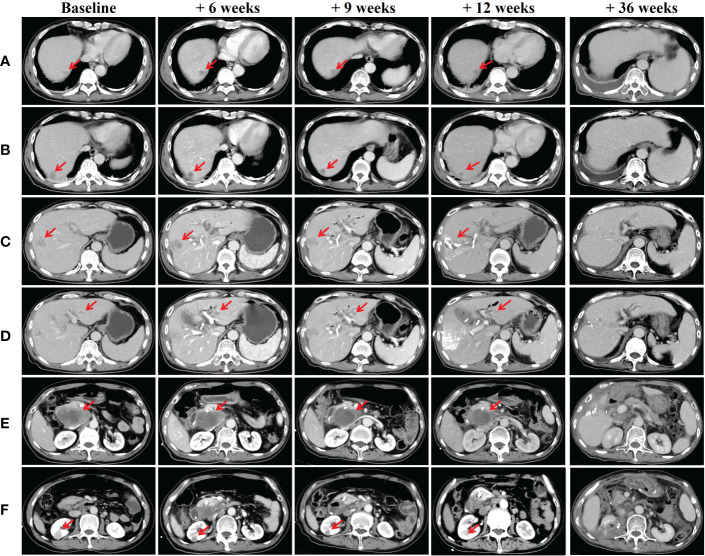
CT scans of the liver, posterior pancreas head and kidneys (A-D) Multiple intrahepatic space-occupying lesions; (E) Mass in posterior pancreas head; (F) Right renal mass. Abdominal CT scans were taken on baseline scan, baseline + 6 weeks (after first session: pembrolizumab and radiotherapy), baseline + 9 weeks (after second session: pembrolizumab plus lenvatinib), baseline + 12 weeks (after third session: pembrolizumab plus TACE) and baseline + 36 weeks (after synchronous partial resection of the liver and partial right nephrectomy).
